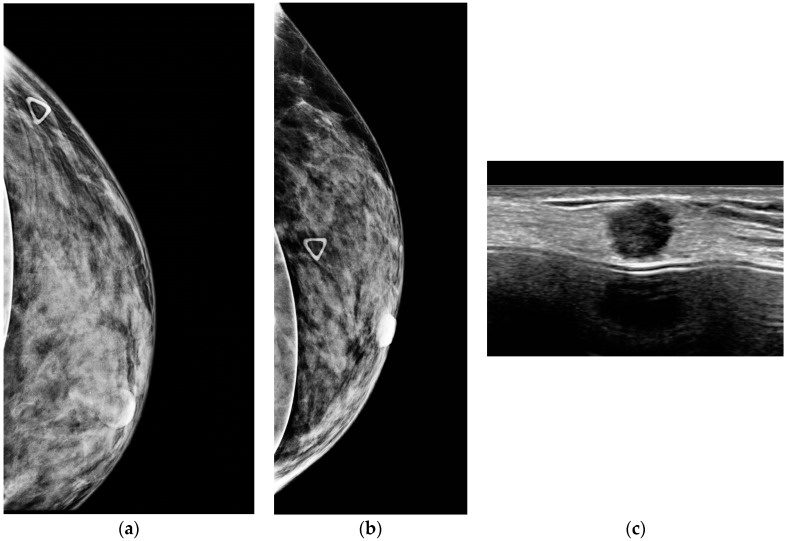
A 53-year-old woman with dense breasts and a palpable abnormality presents for evaluation. (a,b) Implant displaced CC and ML digital mammograms fail to reveal a mass (triangle denotes palpable abnormality). (c) High resolution handheld ultrasound easily depicts a 0.9 cm spiculated mass due to invasive ductal carcinoma.
